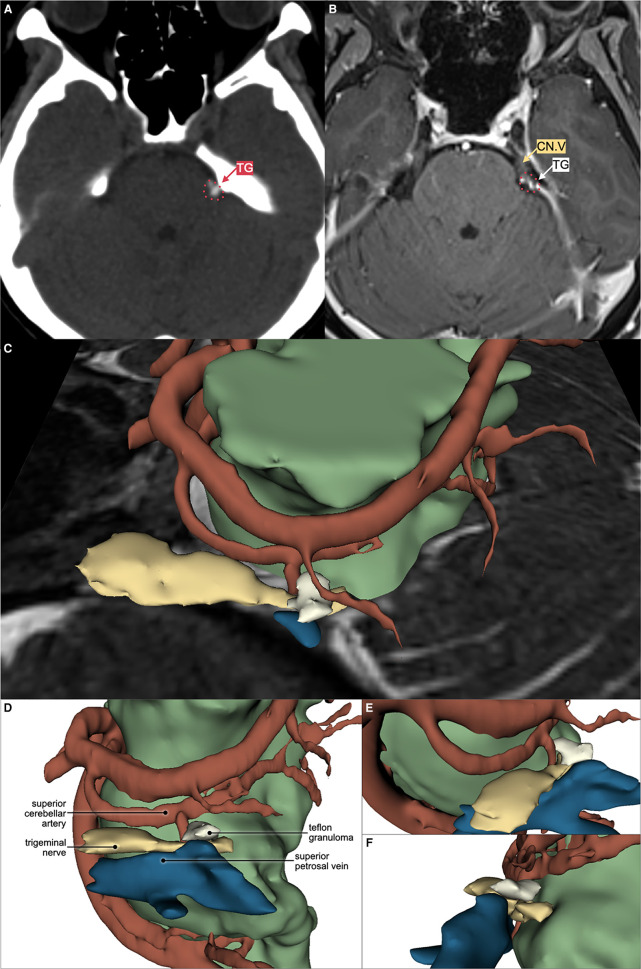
Axial computed tomography (CT) scan without contrast showing hyperdense lesion (A) and magnetic resonance imaging (MRI) T1 with gadolinium contrast (B) showing T1 low to medium intensity, contrast-enhancing lesion compressing the superolateral portion of left trigeminal nerve root entry zone (CN.V), suggesting Teflon granuloma (TG). Left superior (C), left lateral (D), left anterolateral (E), and posterolateral (F) view of the 3D reconstruction using 3D Slicer™ software showing the relation between Teflon granuloma, trigeminal nerve, superior cerebellar artery, superior petrosal vein, and the remaining brainstem parenchyma. Trigeminal nerve is coloured as yellow (
